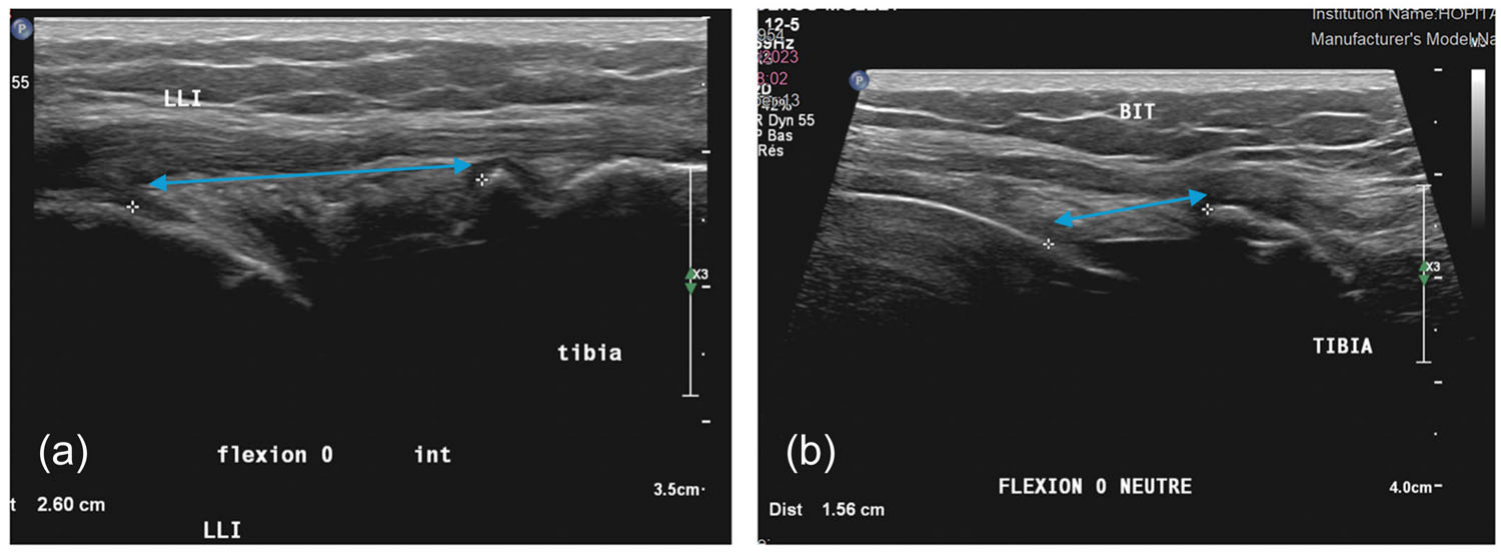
Reference ultrasound landmarks for femorotibial joint space measurement. (a) Typical ultrasound image of the medial joint space. (b) Typical ultrasound image of the lateral joint space. BIT, Iliotibial band; LLI, medial collateral ligament.
